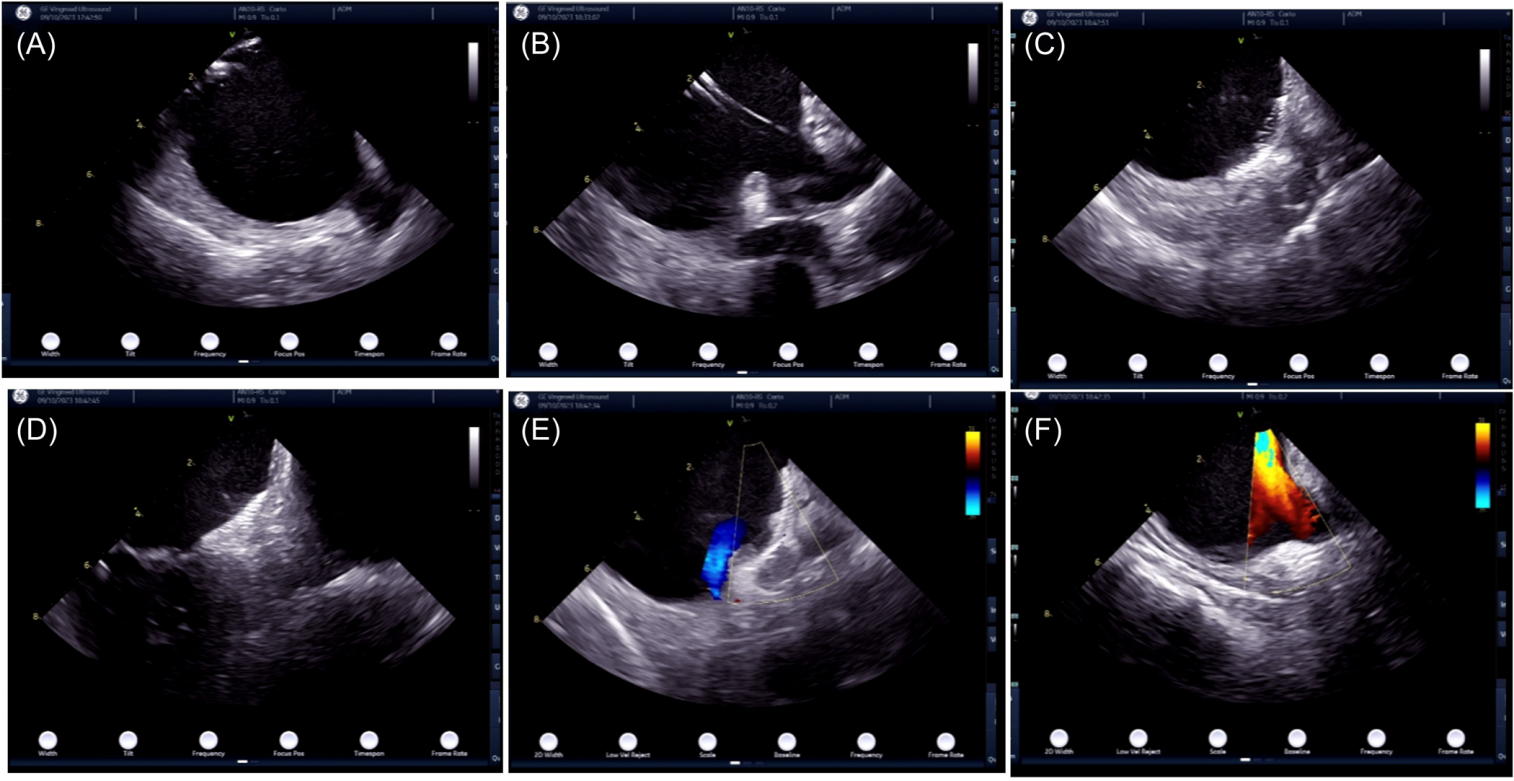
The images of the echo windows in intracardiac echocardiography. (A) Right atrium; (B) right ventricle; (C) left atrium; (D) relationship between left atrium and mitral valve; (E and F) intracardiac blood flow.
